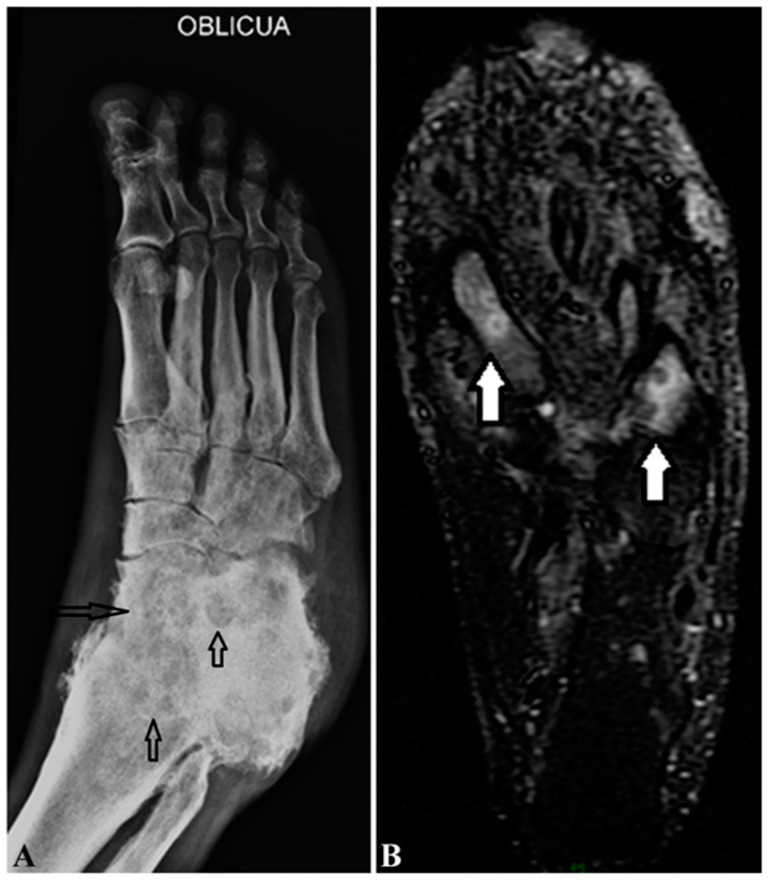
Radiographs of actinomycetoma on a foot. (A) Oblique radiograph of actinomycetoma on the right foot revealing multiple, mixed punched-out (lytic and blastic) cavities (black hollow arrows) of the tarsus and obliteration of joint margins. (B) Coronal MRI view in STIR sequence of the left foot of a 40-year-old male with actinomycetoma showing at least two lesions in the first and fourth metatarsal bones with “dot in circle” signs (white solid arrows) characterized by a hyperintense circle in T2-weighted sequences with a hypointense center. Courtesy of Dr. Jesus Dante Guerra.
